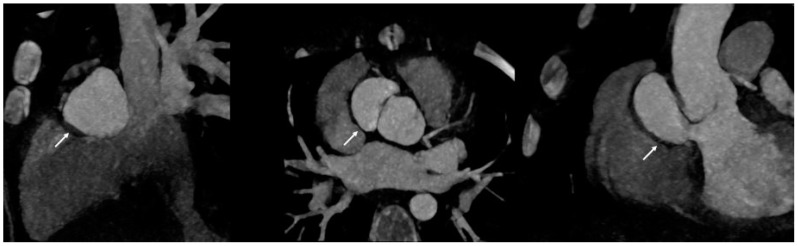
Cardiac CT of a 6-year-old girl with infective endocarditis due to *Kingella kingae* complicated by a paraaortic pseudoaneurysm (white arrow).
